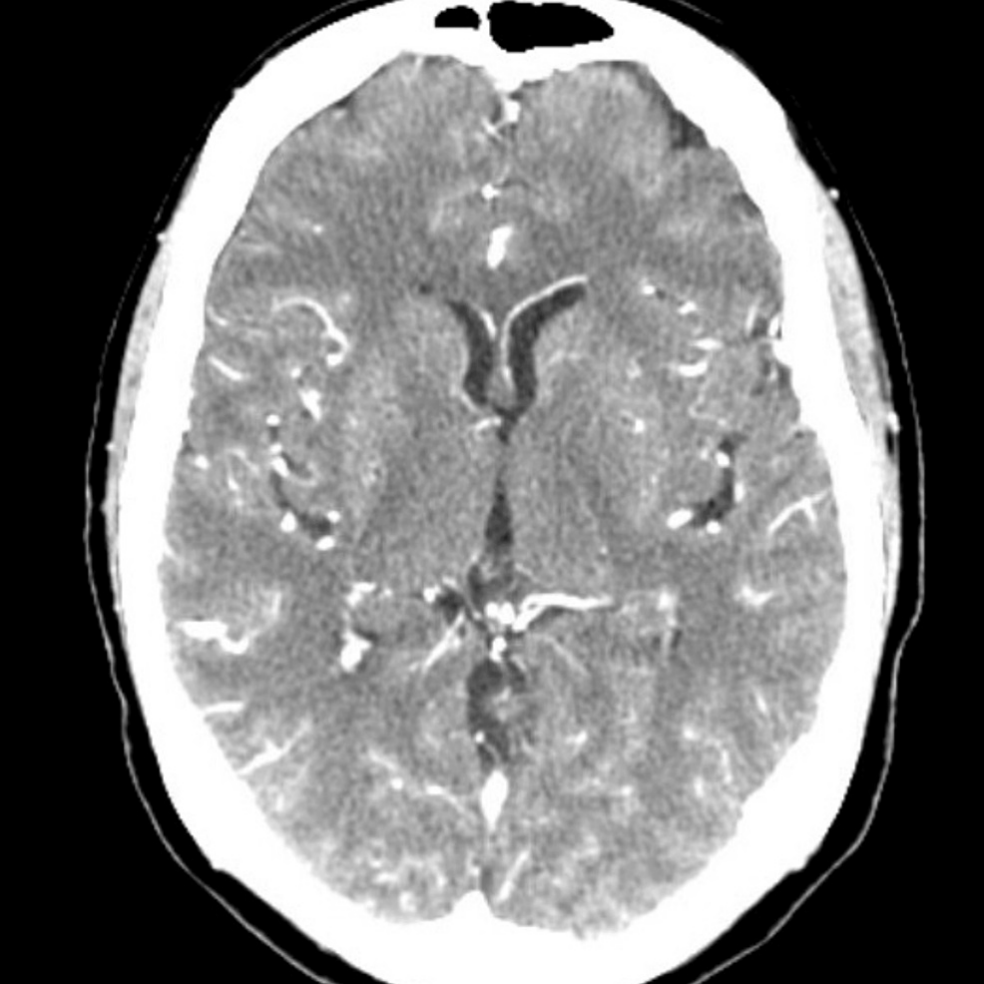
CT angiography head with and without contrast: no acute findings.
